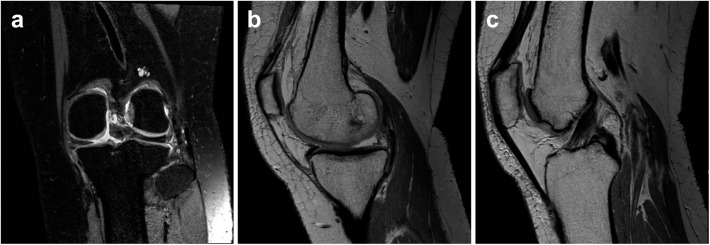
Knee MRI of a patient who had a medial meniscus root tear. Fat-saturated proton-density-weighted images in the coronal scan (a) and proton-density-weighted sagittal (b) scan showed a defect in the medial meniscus posterior horn root. A medial extrusion of the meniscus and high-grade chondral lesions due to osteoarthritis are noted. A proton-density-weighted sagittal scan at a different level (c) showed an ACL that was intact.
